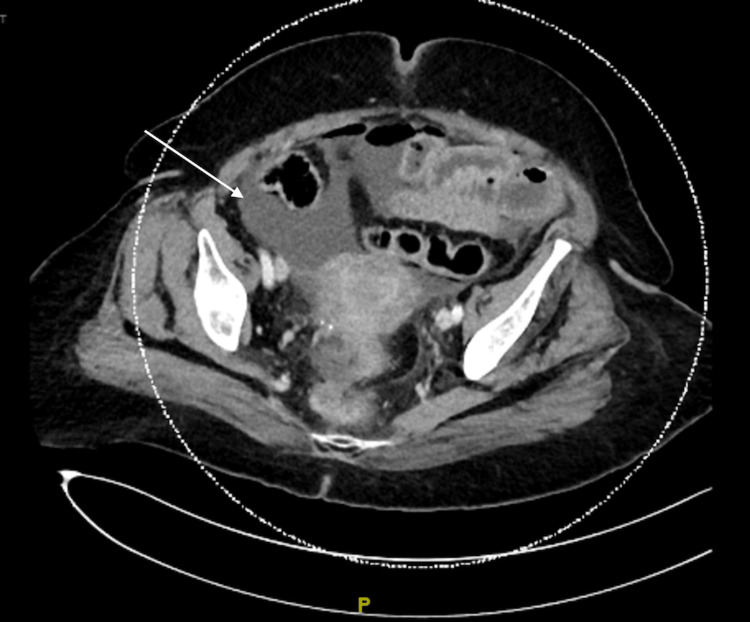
Abdominopelvic CT scan showing moderate peritoneal fluid (white arrow).
